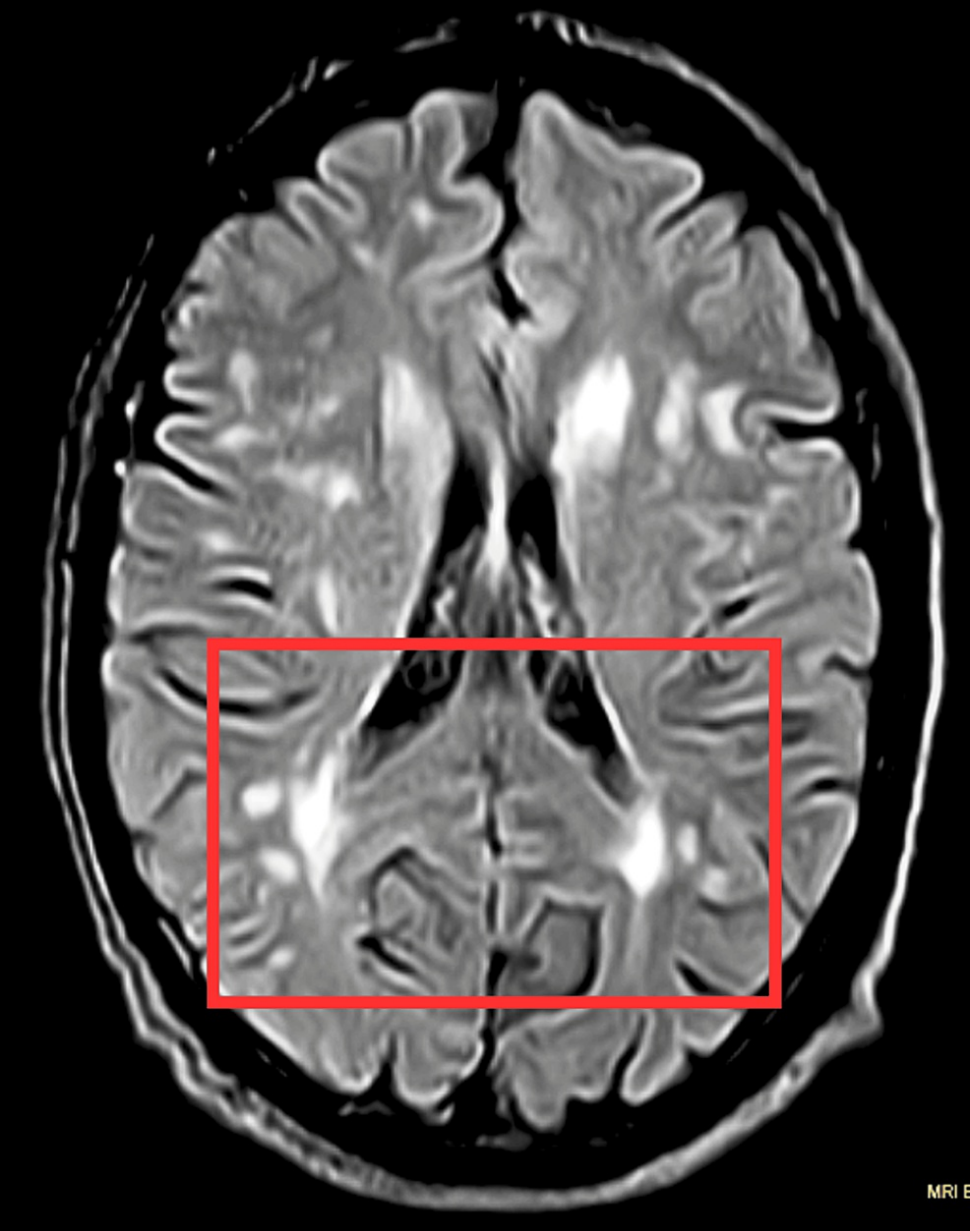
MRI brain The red rectangle represents bilateral pontine infarct. MRI, magnetic resonance imaging
